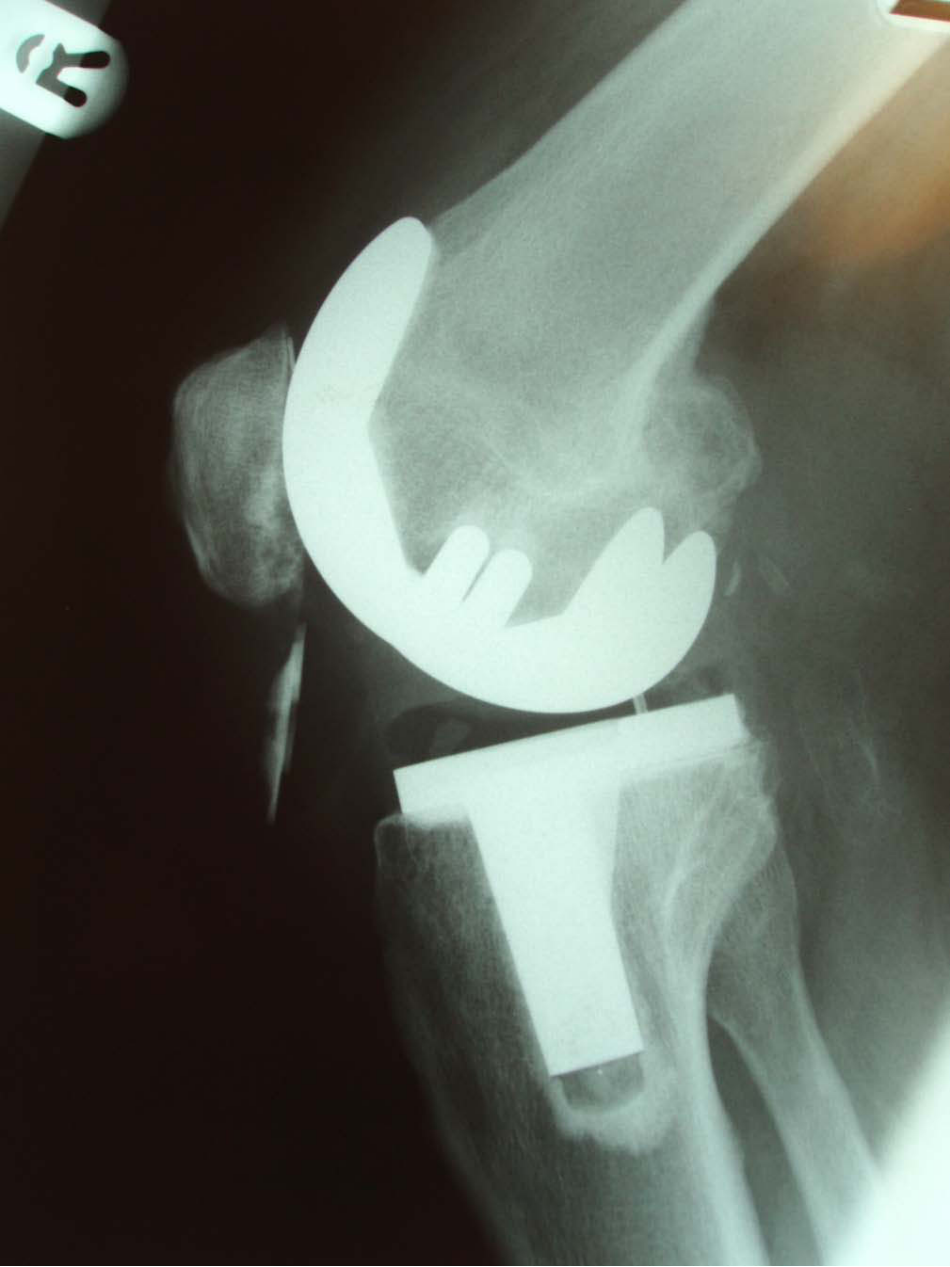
Sagittal radiograph of the knee showing dislocated patellar prosthesis.
